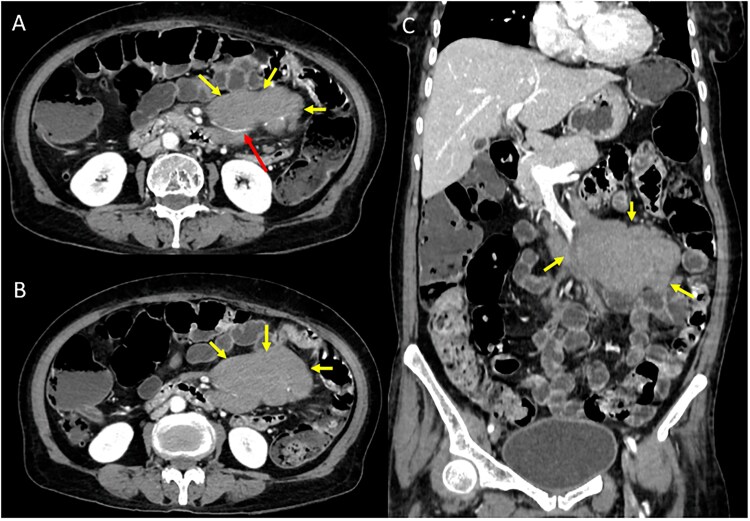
Contrast-enhanced Computed Tomography (CECT) axial (A,B) and coronal (C) images reveal intraperitoneal lobulated homogeneously enhancing nodal mass in the mesentery encasing branches of superior mesenteric artery without luminal attenuation giving ‘Sandwich sign’ suggestive of lymphoma.
